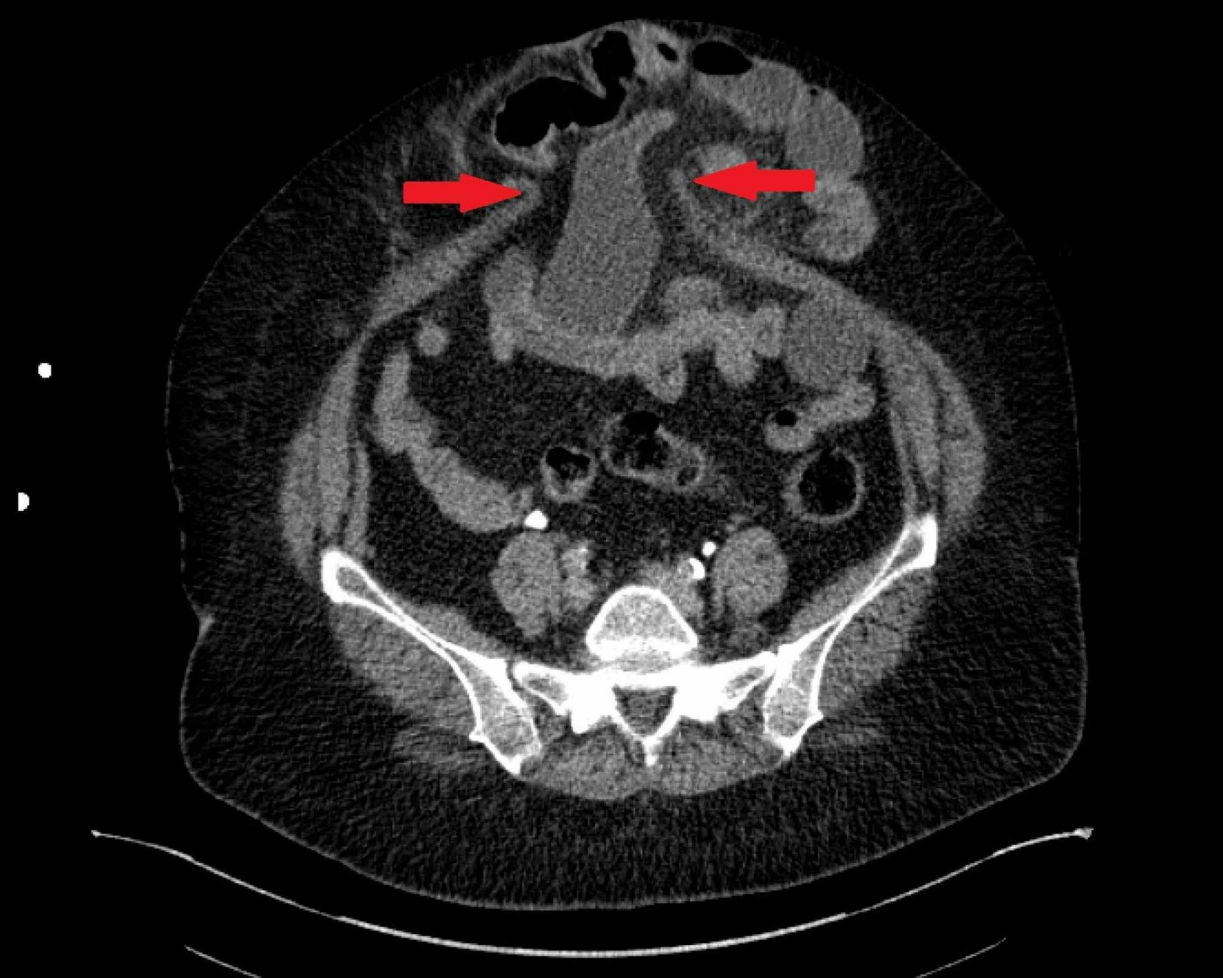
Initial CT scan demonstrating pre-existing ventral hernia (see arrows) with no free air, free fluid, intra-abdominal inflammatory changes, or other signs of perforated viscus
